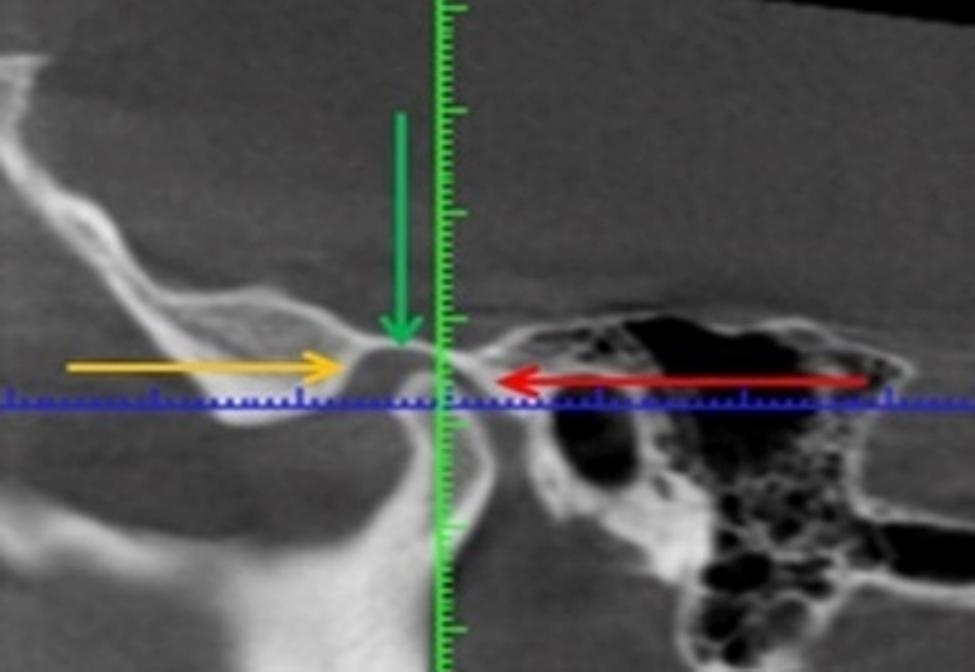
Computer tomography (CT) before treatment
